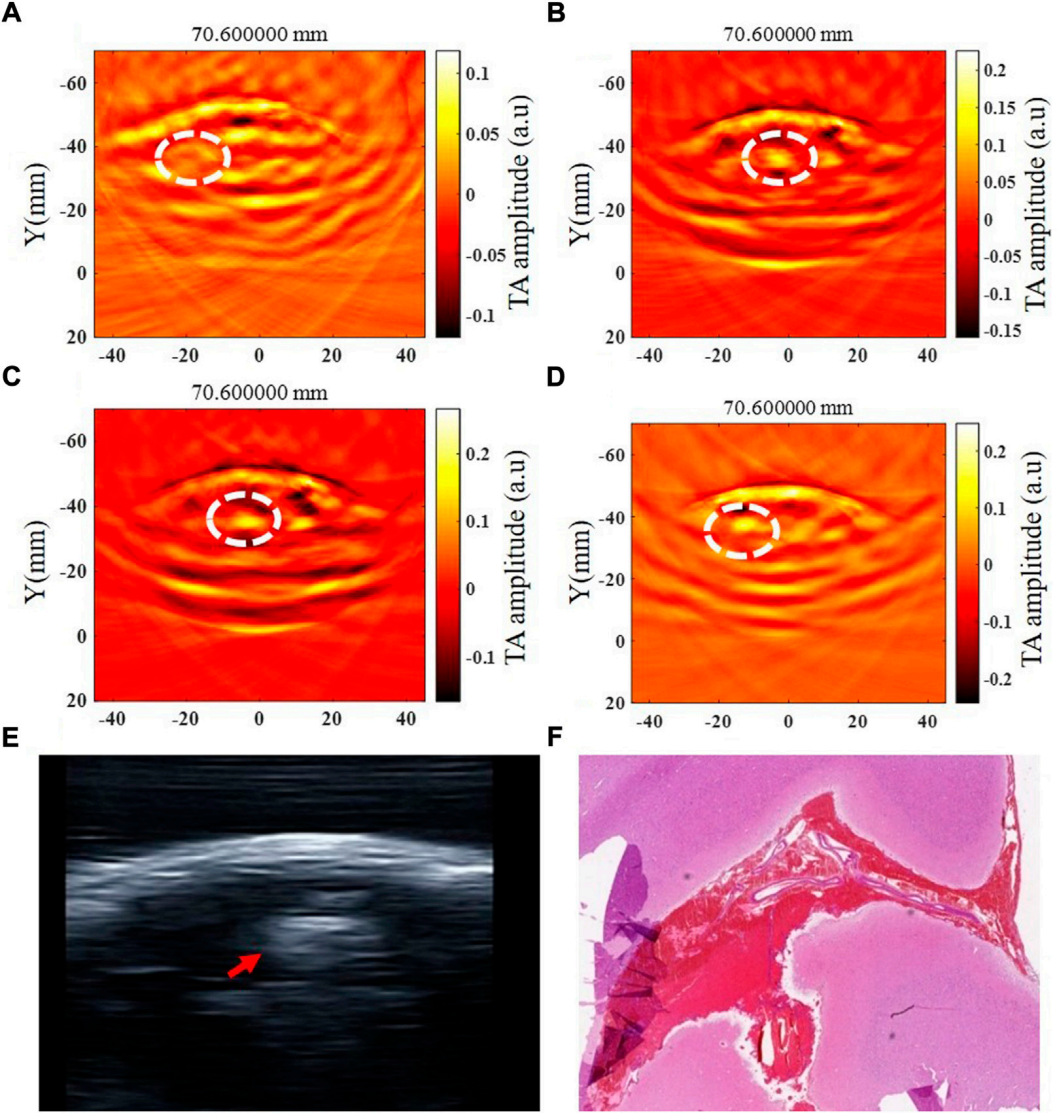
Thermoacoustic imaging, ultrasound and pathological examination of a same canine intracerebral hemorrhage. (A–D) TAI images at 0.5 h, 1 h, 3 h and 6 h after modeling, respectively (white circle shows the hemorrhagic area). (E) Ultrasound image of canine intracerebral hemorrhage (white circle shows the hemorrhagic area). (F) Pathological image of canine intracerebral hemorrhage.
